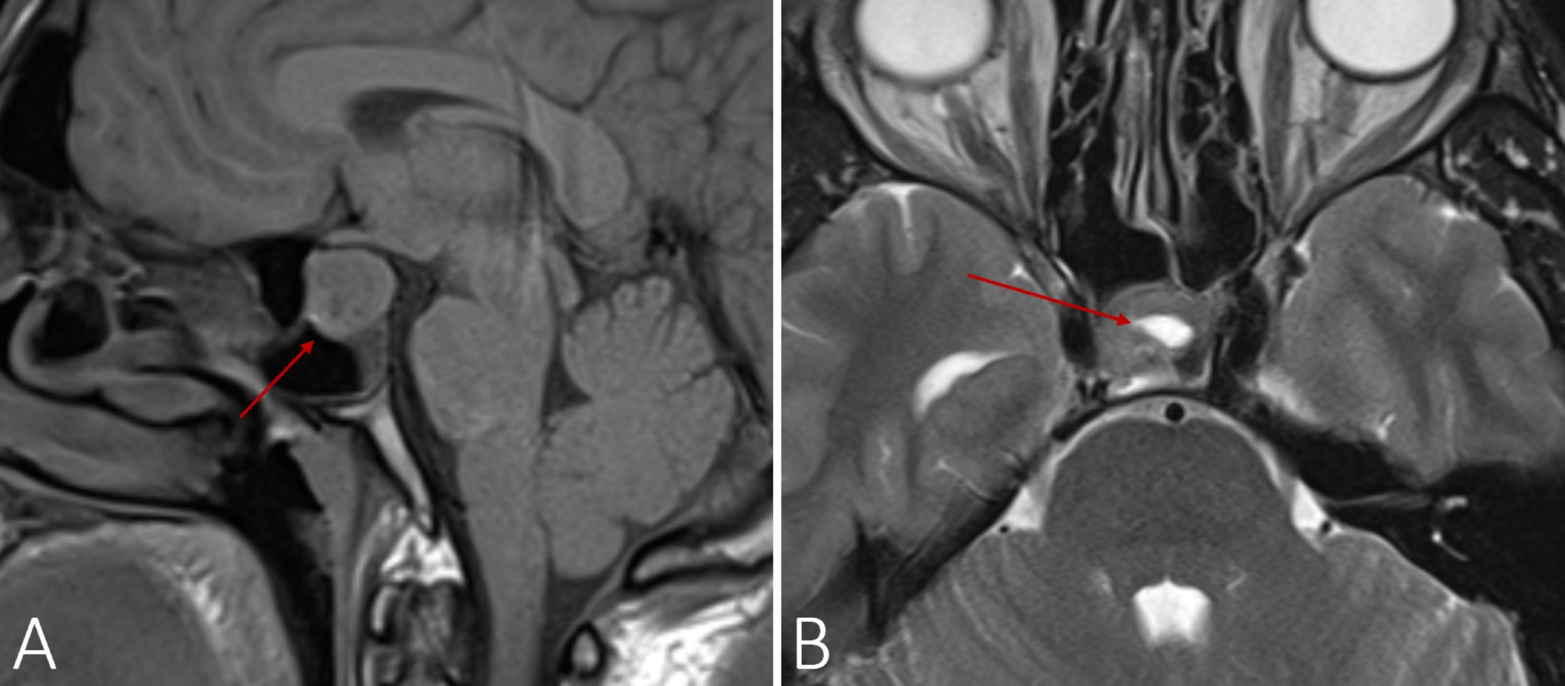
Cranial MRI: T1-weighted saggital plane (A) and T2-weighted axial plane (B) demonstrating a pituitary macroadenoma (214 mm x 168 mm x 147 mm); in the latter image, hyperintense filling (arrow) of the adenoma is also visible, suggesting pituitary apoplexy.
